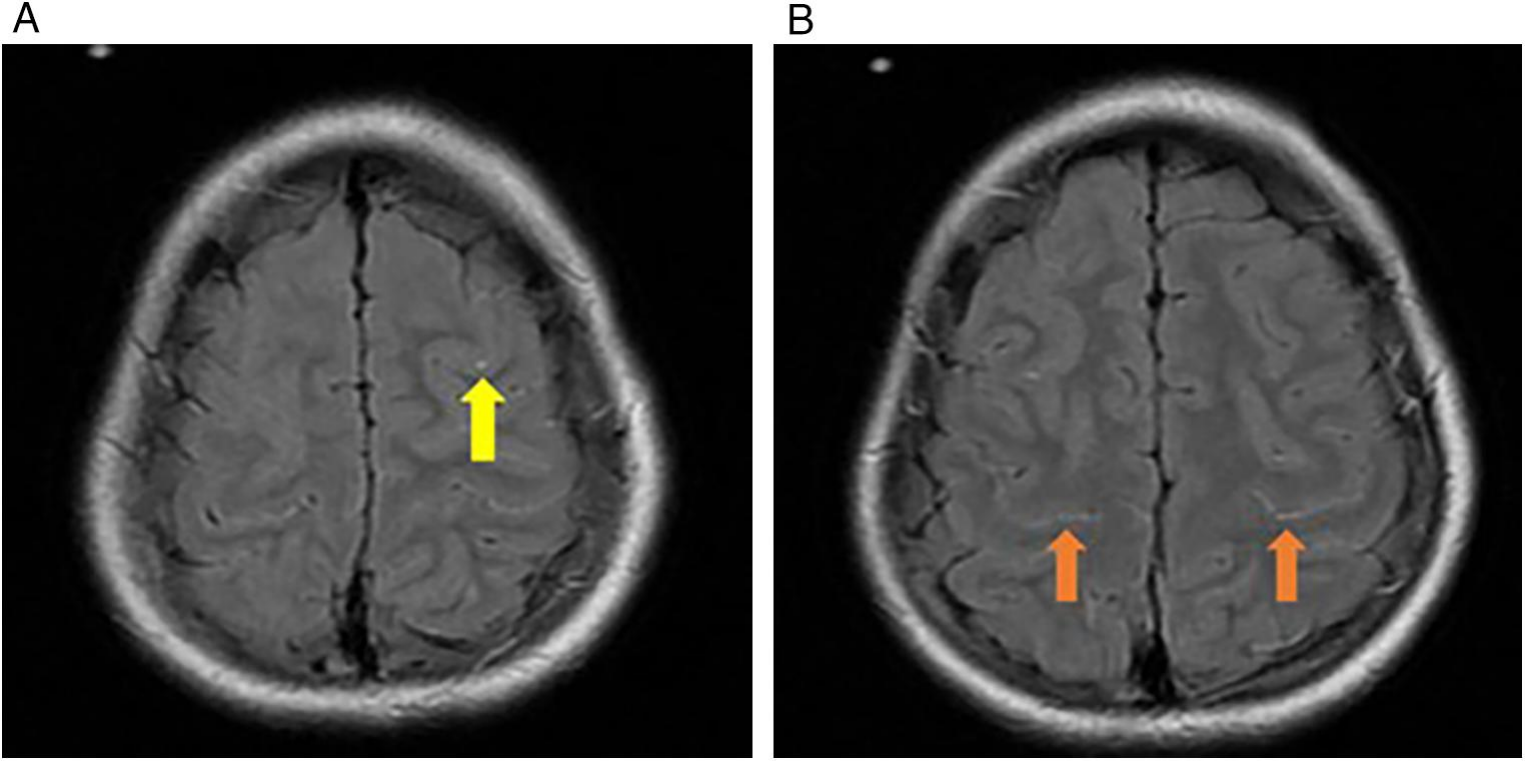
(A) punctate FLAIR slightly high, signal shadows observed in the frontal lobe sulci (yellow arrow); (B) linear FLAIR slightly high, signal shadows are observed in the bilateral parietal lobe sulci (orange arrows).
